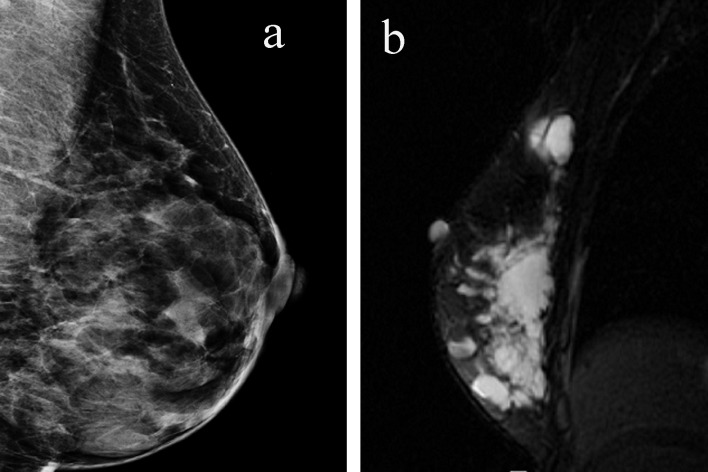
(a) Mammogram reveals multiple cystic lesions. (b) MRI shows the entire right breast is filled with multiple cystic structures with internal loculations.
